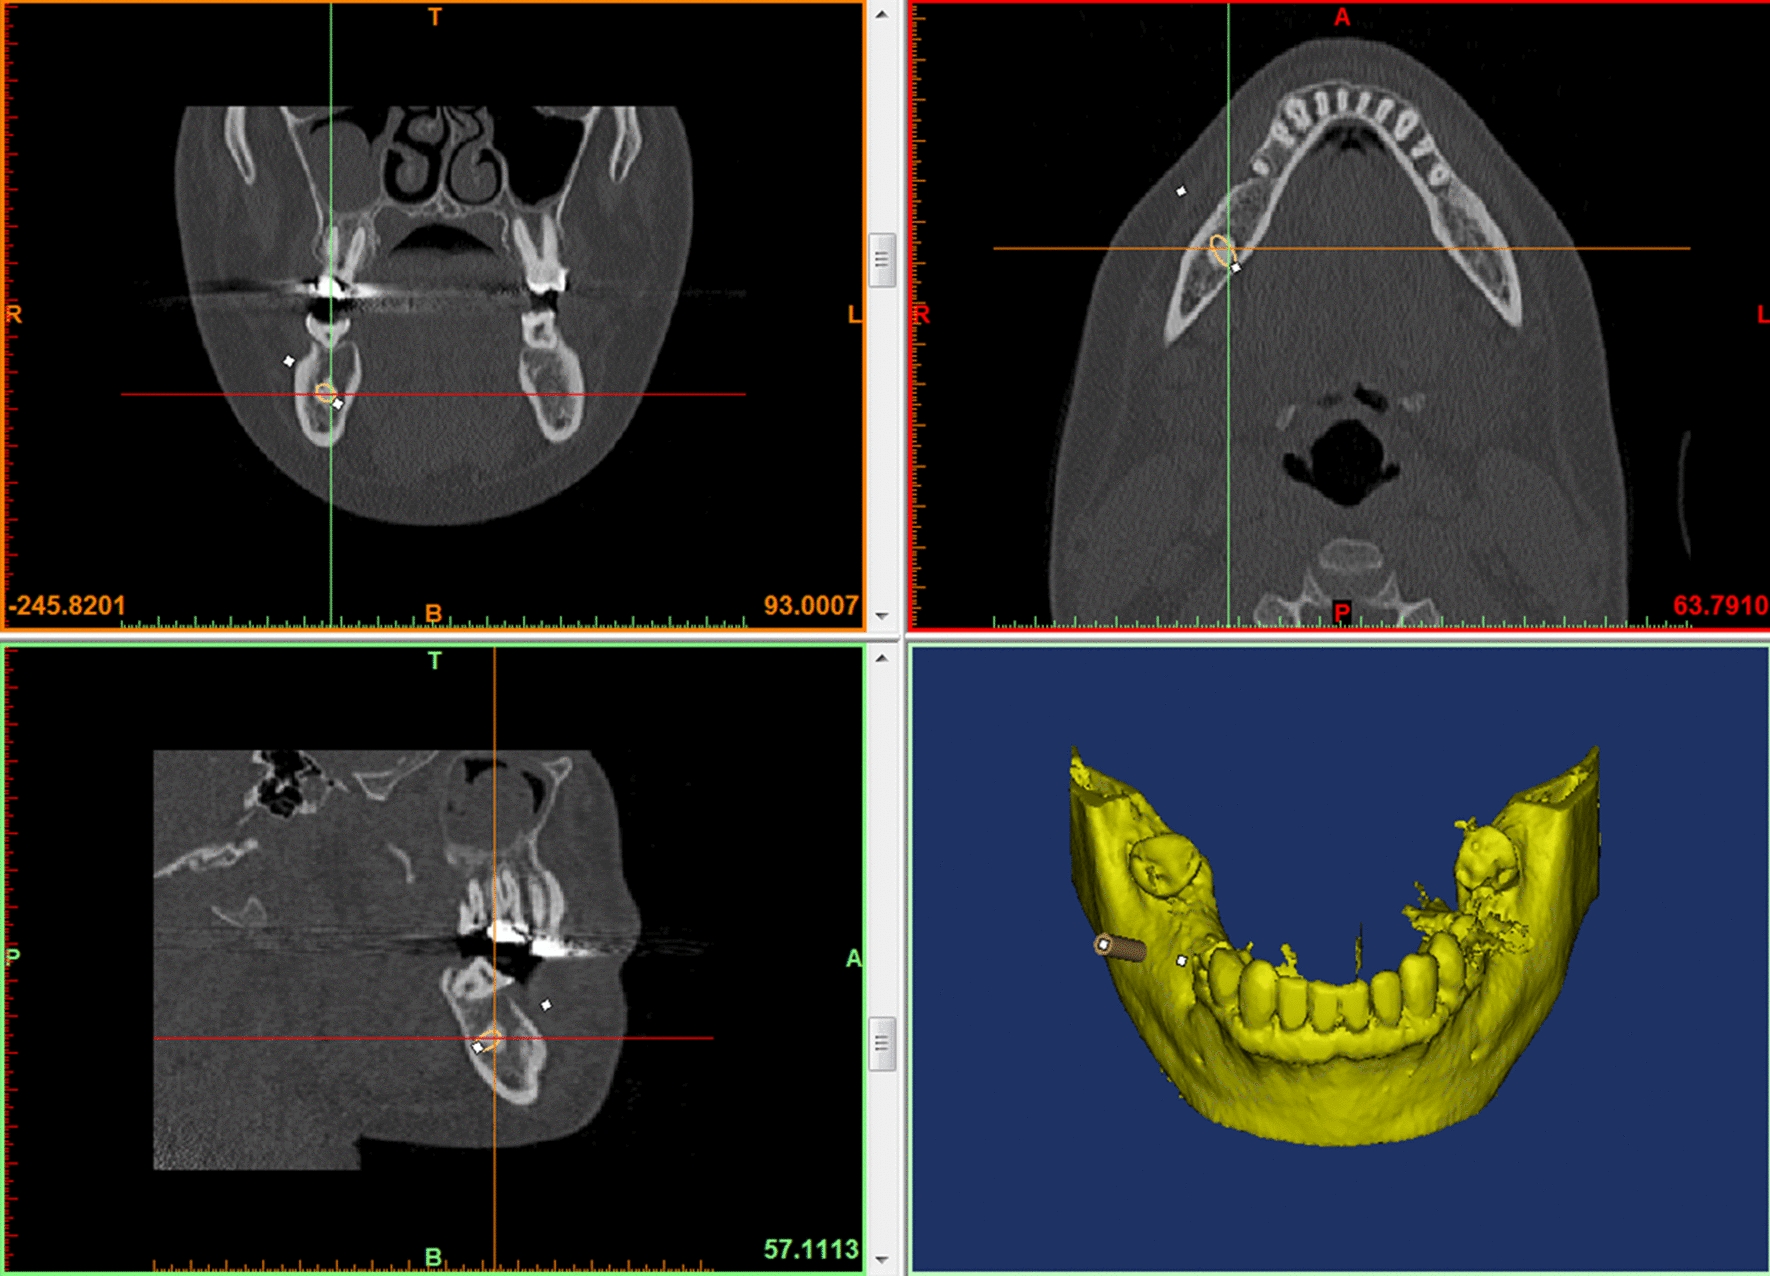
Part of a screenshot while planning of the biopsy channel according to CT data
